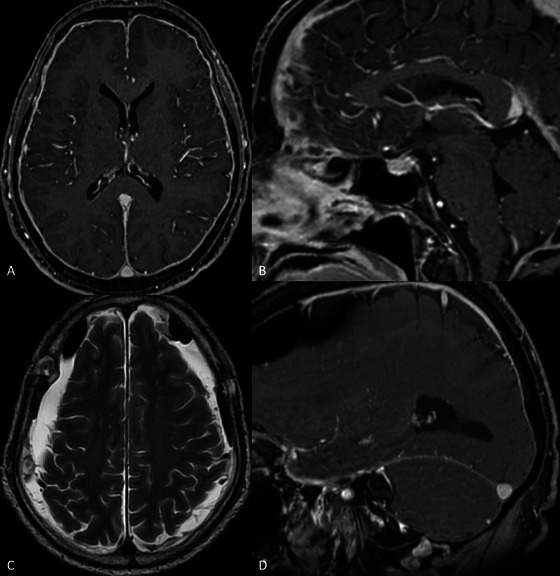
Brain magnetic resonance imaging (MRI) showing signs of intracranial hypotension. (Panel A) Axial post‐contrast 3D FSPGR image showing diffuse pachymeningeal enhancement, a hallmark sign of intracranial hypotension due to compensatory meningeal vascular engorgement. (Panel B) Sagittal post‐contrast 3D FSPGR image demonstrating signs of brain sagging, with reduction of the mammillopontine distance, as well as effacement of the prepontine and suprasellar cisterns. (Panel C) Axial T2‐weighted image showing bilateral subdural HYPERINTENSE fluid collections with air‐fluid levels following surgical evacuation via fronto‐parietal burr holes. (Panel D) Sagittal post‐contrast 3D FSPGR image depicting engorgement of the right transverse sinus, reflecting venous distension secondary to low intracranial pressure.
